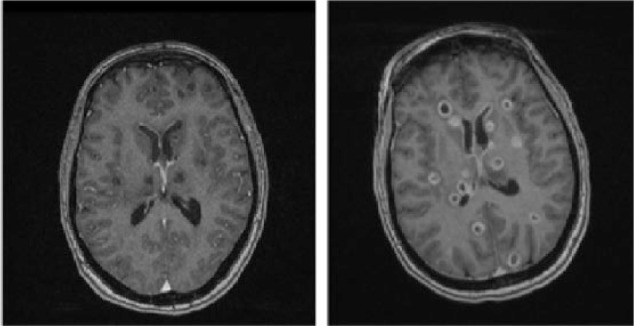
Contrast MRI on left from day of admission shows little if any peripheral enhancement. Image on right, obtained 9 days later, shows interval organization of parenchymal abscess with rim enhancement.
